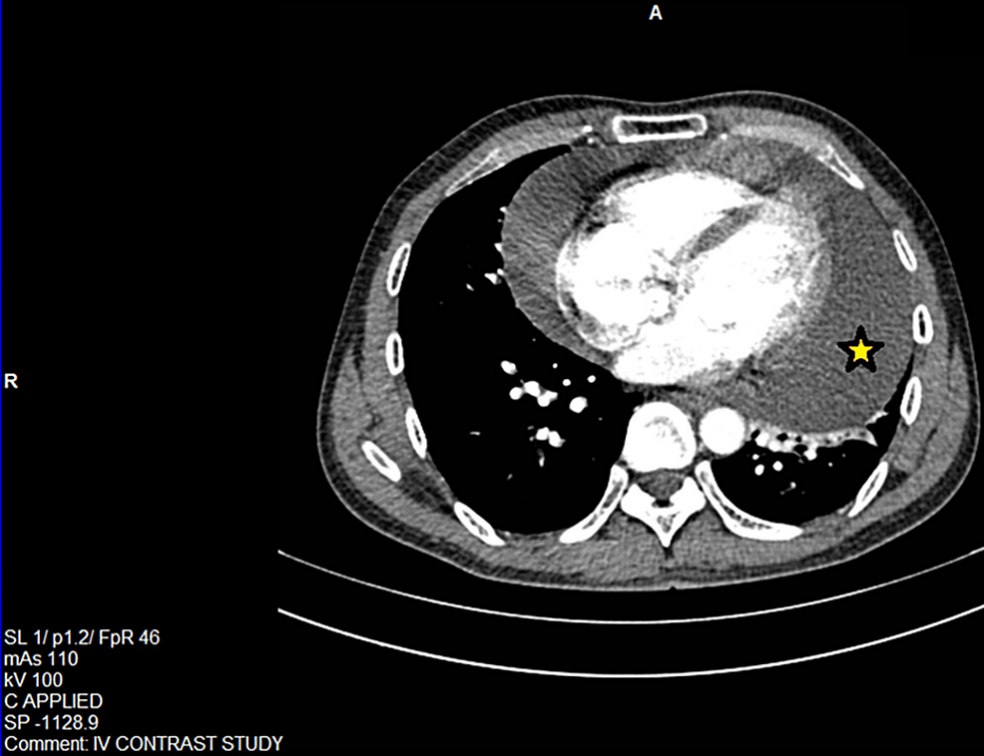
Axial contrast-enhanced CT in arterial phases at a mid-thoracic level in the mediastinal window showing normal size cardiac chambers with gross pericardial effusion (asterisk)
